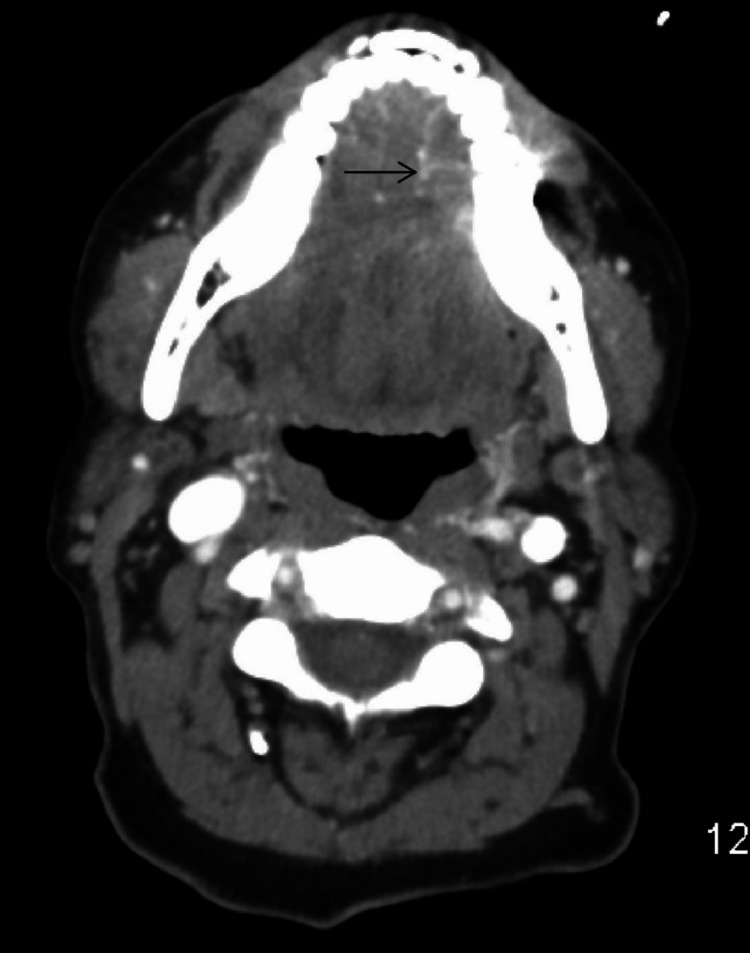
A 57-year-old female with moderately differentiated oral squamous cell carcinoma. Axial contrast-enhanced CT image demonstrates heterogeneously enhancing mass in the left lateral tongue with peripheral enhancing capsule and mild medial displacement of the neurovascular bundle. CT: computed tomography
